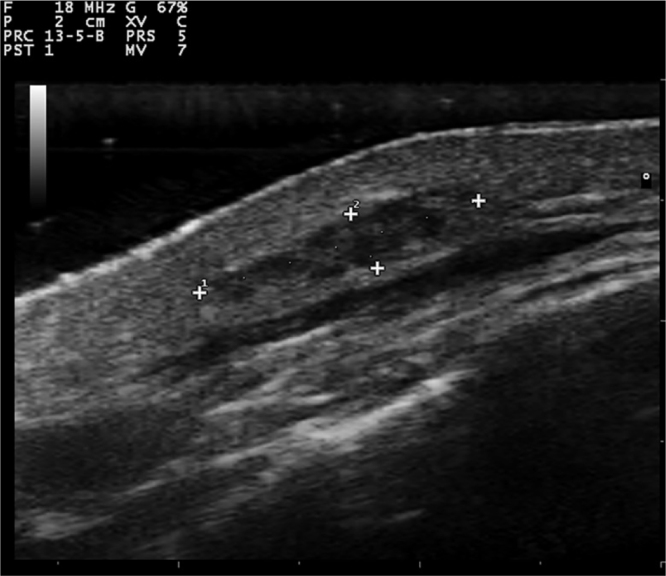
Transverse sonogram (18 MHz) of the subcutaneous myxoma, showing an ill-defined, elongated, heterogeneous, and hypoechoic mass located in the deep dermis and hypodermis.
